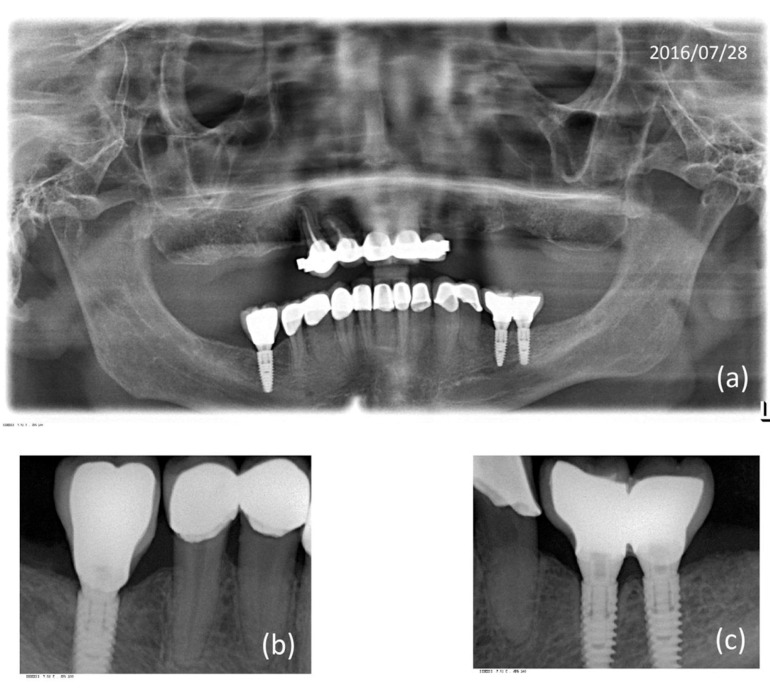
Radiographic control dated July 28, 2016. (a) OPG with fixed prosthesis (b) Rehabilitation implant zone 46. (c) 36-37 Implants zone. 1.25 mm of bone loss is observed in the implant 36.
